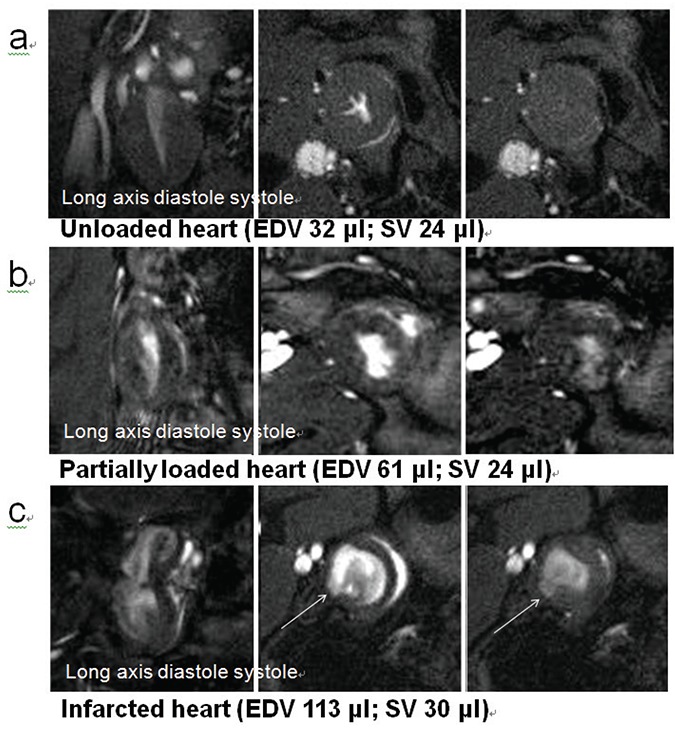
a, MRI cine frames (long axis; mid-ventricular short axis diastole; mid-ventricular short axis systole, slice frame 1.5mm; EDV: end-diastolic volume; SV: stroke volume) showing unloaded left ventricle; coronary venous effluent visible in right ventricle; b, as a, demonstrating partially loaded left ventricle; c, as a, demonstrating regional left ventricular wall motion abnormality (arrow) and paradoxical movement. SV calculated as EDV-ESV.
